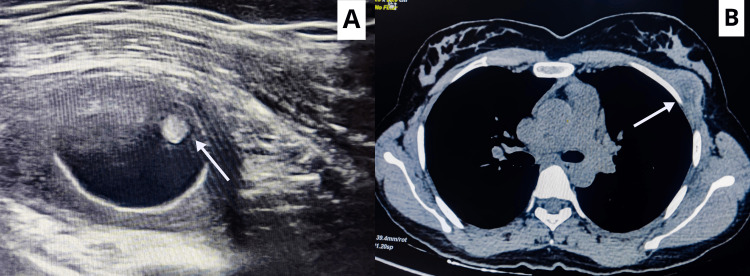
(A) Ultrasonography of the left breast reveals a well-defined oval cyst within a collection and a brightly echogenic protrusion from the wall. (B) A contrast-enhanced computed tomography film showing a cystic lesion in the left anterolateral chest wall in the intermuscular plane of the pectoralis minor muscle.
